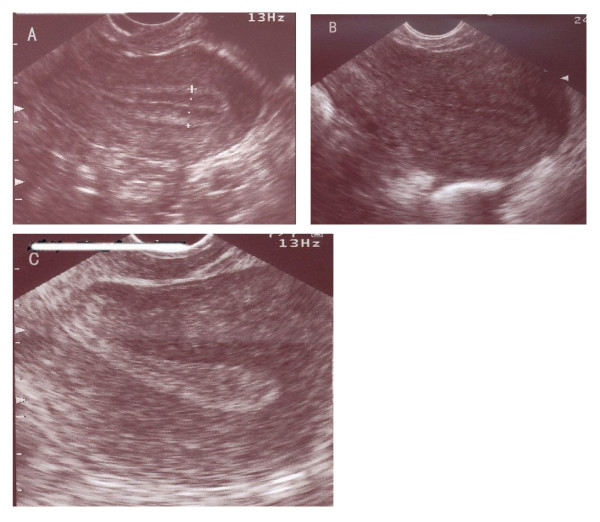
Longitudinal ultrasound images demonstrate the endometrial pattern. (A) Triple-line pattern: hypoechoic endometrium with well-defined hyperechoic outer walls and a central echogenic line; (B) No-triple line pattern: isoechoic endometrium with poorly defined outer walls and central echogenic line; (C) No-triple line pattern: homogeneous hyperechoic endometrium.
